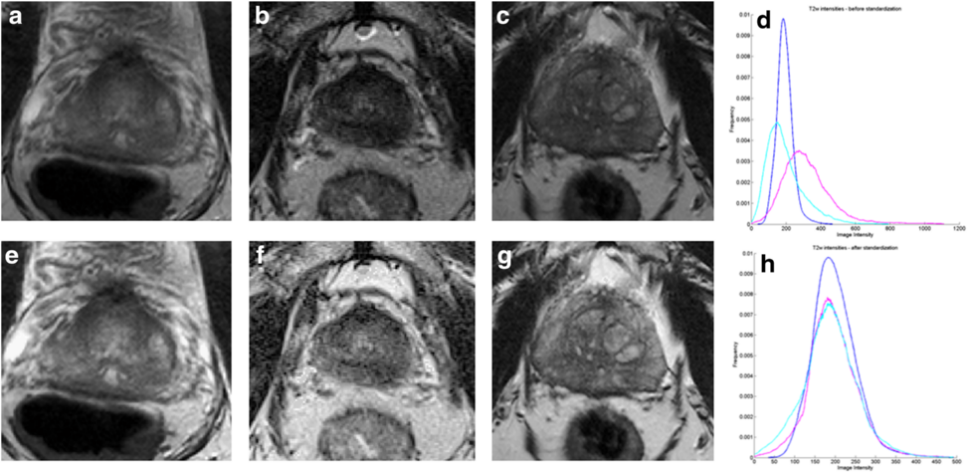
a, b, c show the original T2w MRI images for three patient studies from *D*
